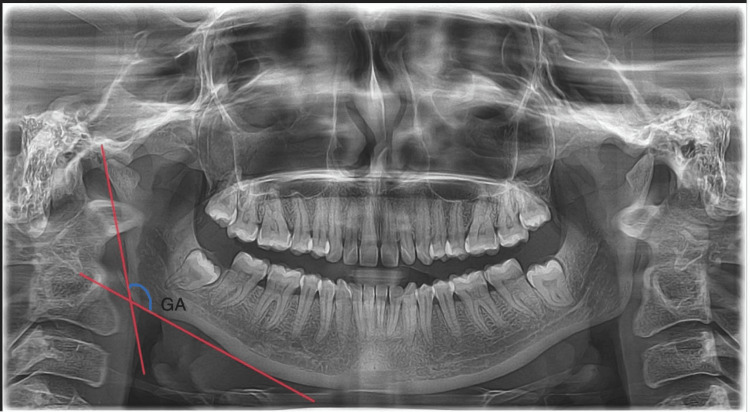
Given radiograph represents the gonial angle formed between the posterior border and inferior border of the mandible
